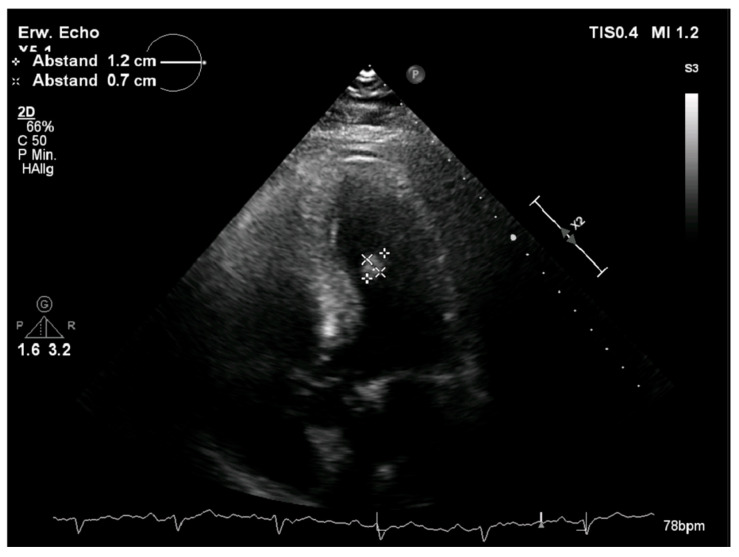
Preoperative echocardiographic examination of the left ventricle. The tumor is measured and marked with white crosses.
